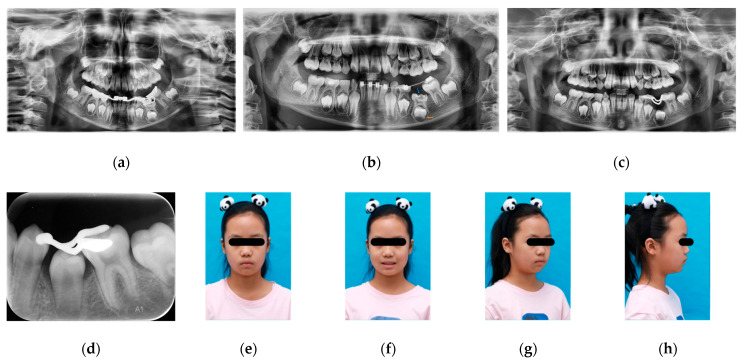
Panoramic radiograph, periapical radiograph and facial photograph during the treatment. (a) Panoramic radiograph obtained at treatment initiation. (b) Panoramic radiograph obtained after 8 months of treatment, showing a radiolucency within the crown of tooth 75 extending to the deep layer of dentin (blue arrow), and the root of tooth 35 reaching Nolla stage VI (orange arrow). (c) Panoramic radiograph obtained after 12 months of treatment, demonstrating uprighting of teeth 74 and 36, with no bony coverage above tooth 35. (d) Periapical radiograph obtained after 31 months of treatment, demonstrating the root development of tooth 35 at Nolla stage VII–VIII. (e–h) Facial photographs obtained after 33 months of treatment.
